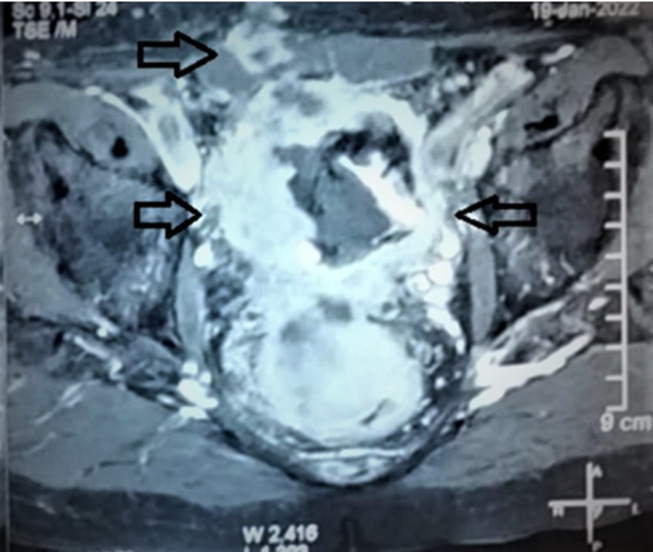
magnetic resonance imaging of the pelvis showing the lesion invading the pubic bone, the right lateral pelvic wall, and the pre-muscular subcutaneous fat (arrows)
